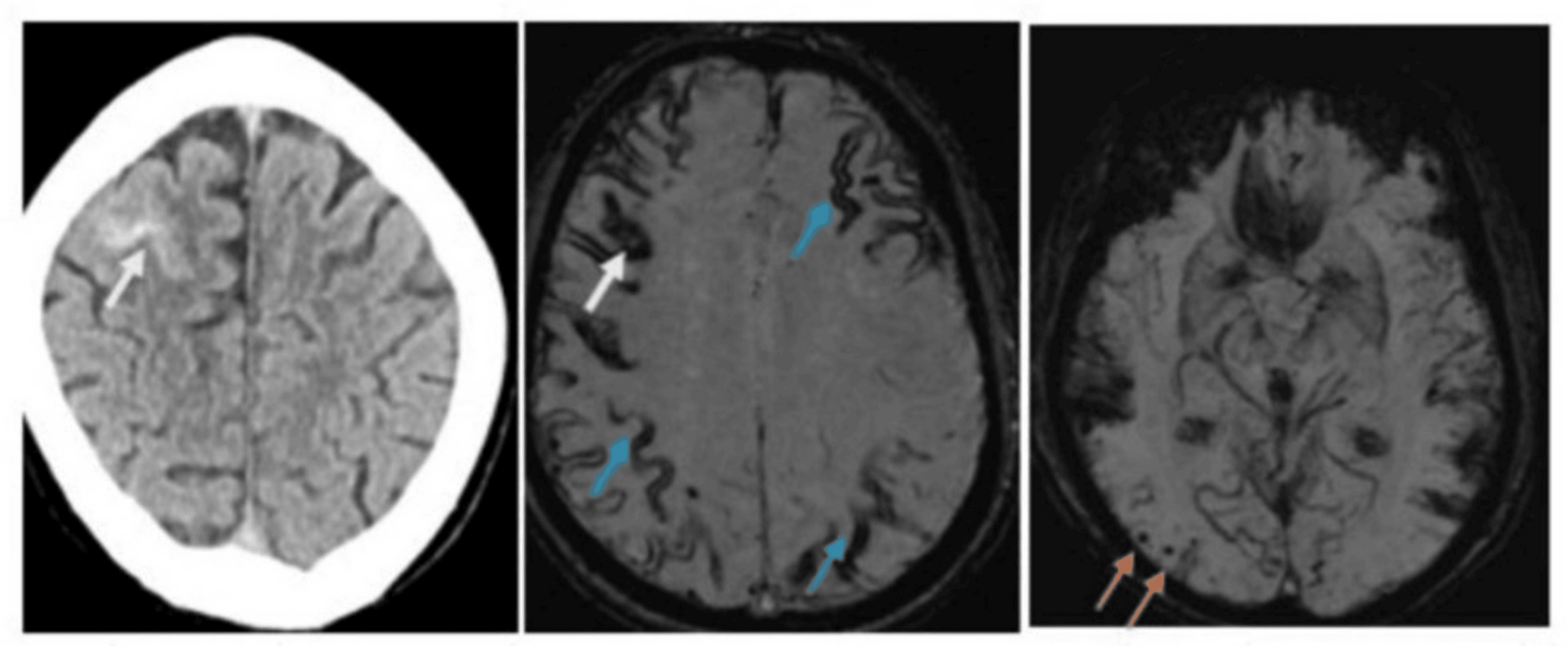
Convexity subarachnoid haemorrhage on non-contrast brain CT confirmed on susceptibility weighted MRI (white arrows) with the presence of cortical siderosis (blue arrows) and multiple lobar microhaemorrhages (orange arrows)
